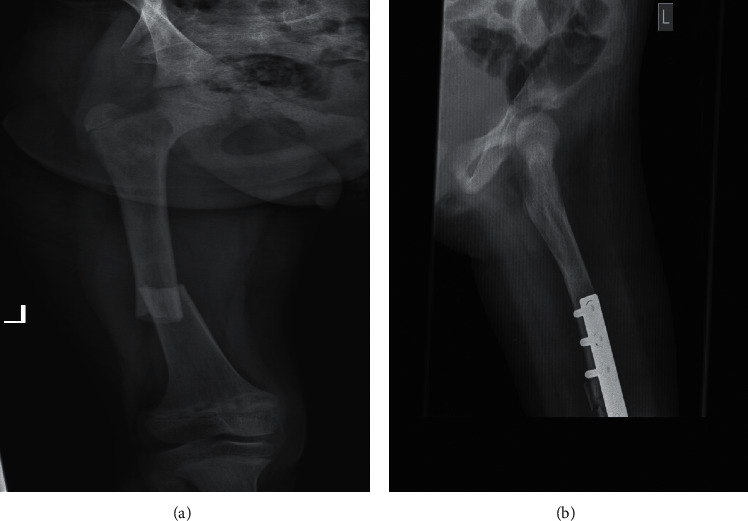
Radiologic appearance of fibrous dysplasia. (a) Left femur X-ray: there was intramedullary expansive lesion resulted in endosteal scalloping with ground glass and lucent matrix involving the femoral neck up to the midlower shaft of the femur. Pathological fracture is seen at the midlower shaft of the femur with overlapping fracture segments. (b) Posttreatment X-ray: callus formation seen at the site of the pathologic fracture.
